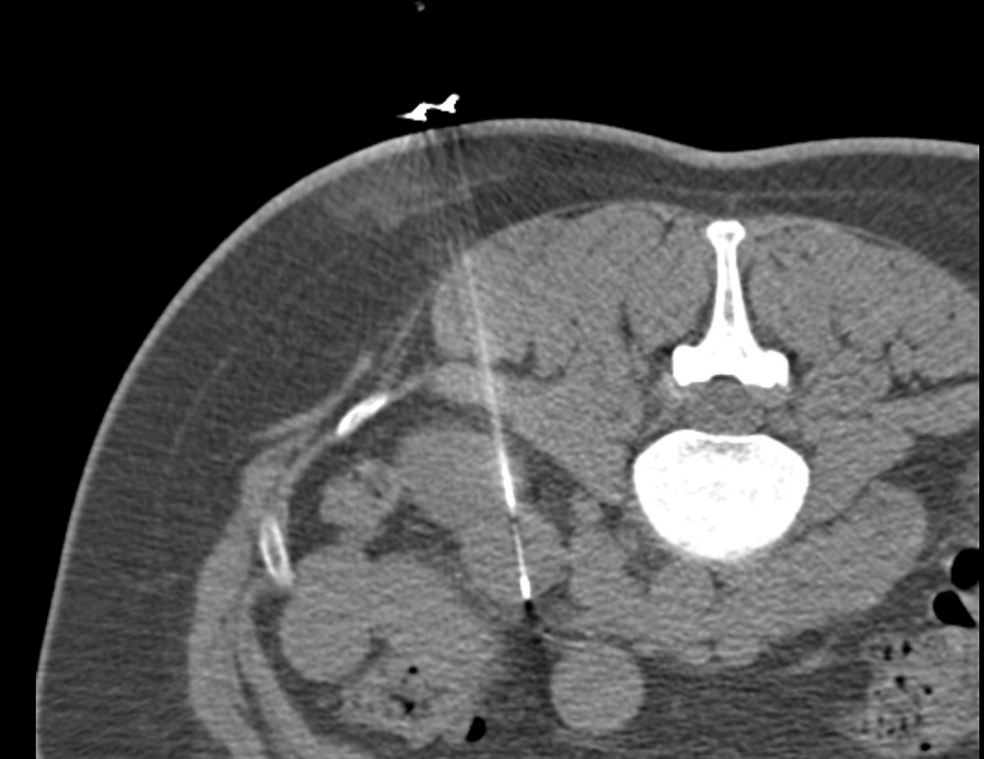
A CT-guided core needle biopsy of the left renal lesion
